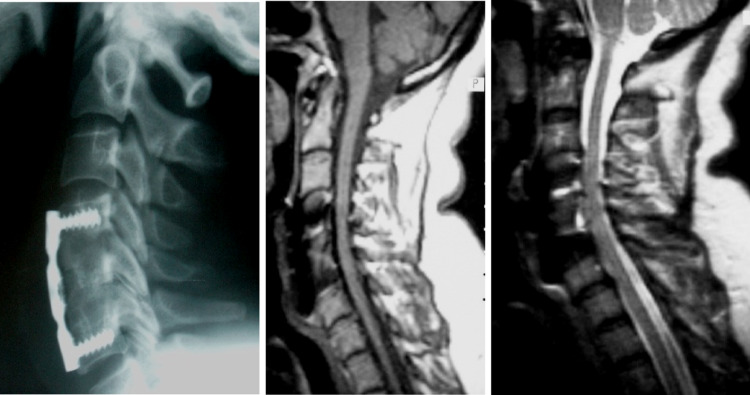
Postoperative lateral cervical spine X-ray showing removal of osteophytes and fusion of the cervical spine from C4 to C6 (a). Postoperative sagittal T1- and T2-weighted MRI images demonstrating evidence of spinal cord decompression (b, c)
